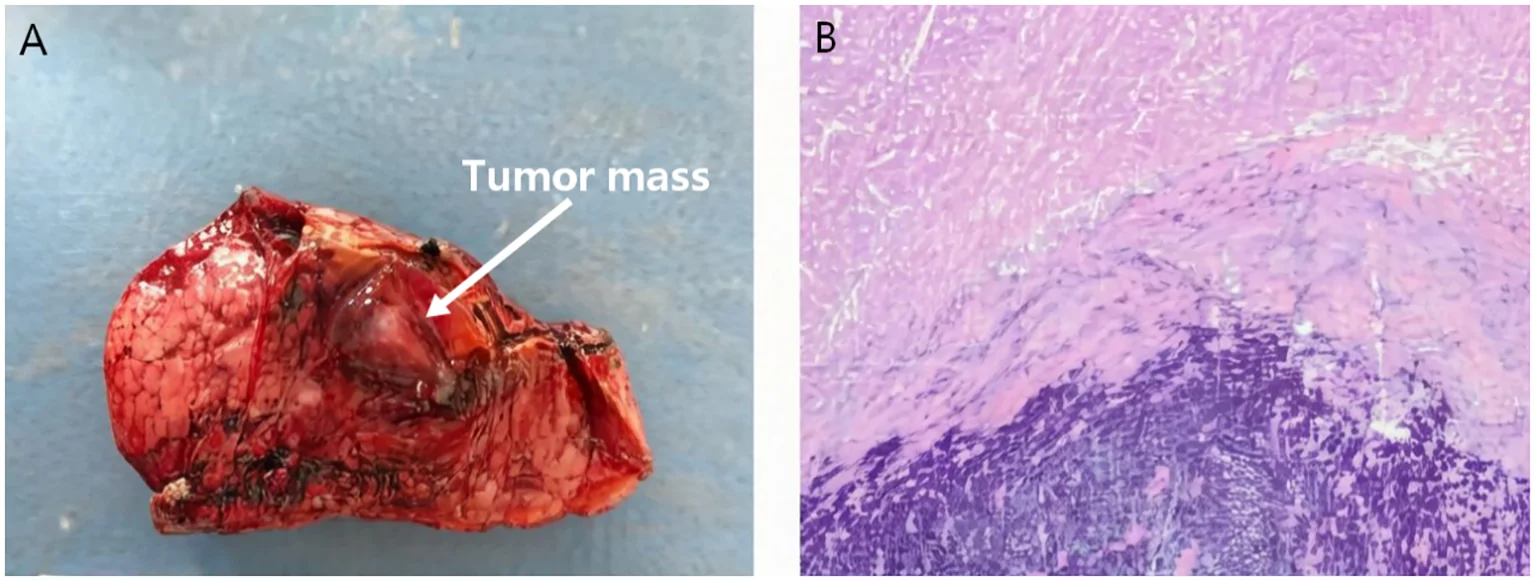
Preoperative axial chest CT series. Preoperative axial chest CT images (lung and mediastinal windows) demonstrate an approximately 5-cm, lobulated, heterogeneous soft-tissue mass adjacent to the left pulmonary hilum.
